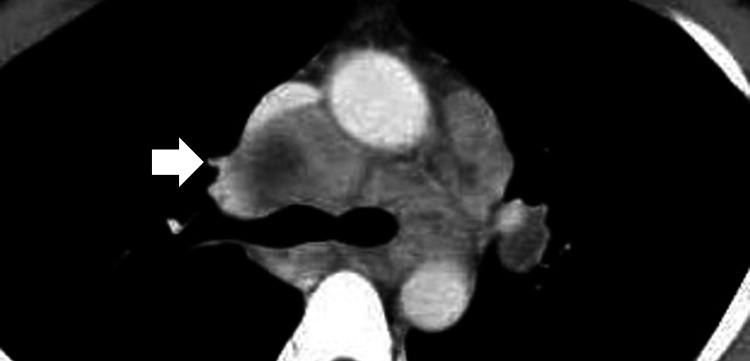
Computed tomography scanning of the chest revealing bilateral hilar and longitudinal lymphadenopathy at the first visit.
